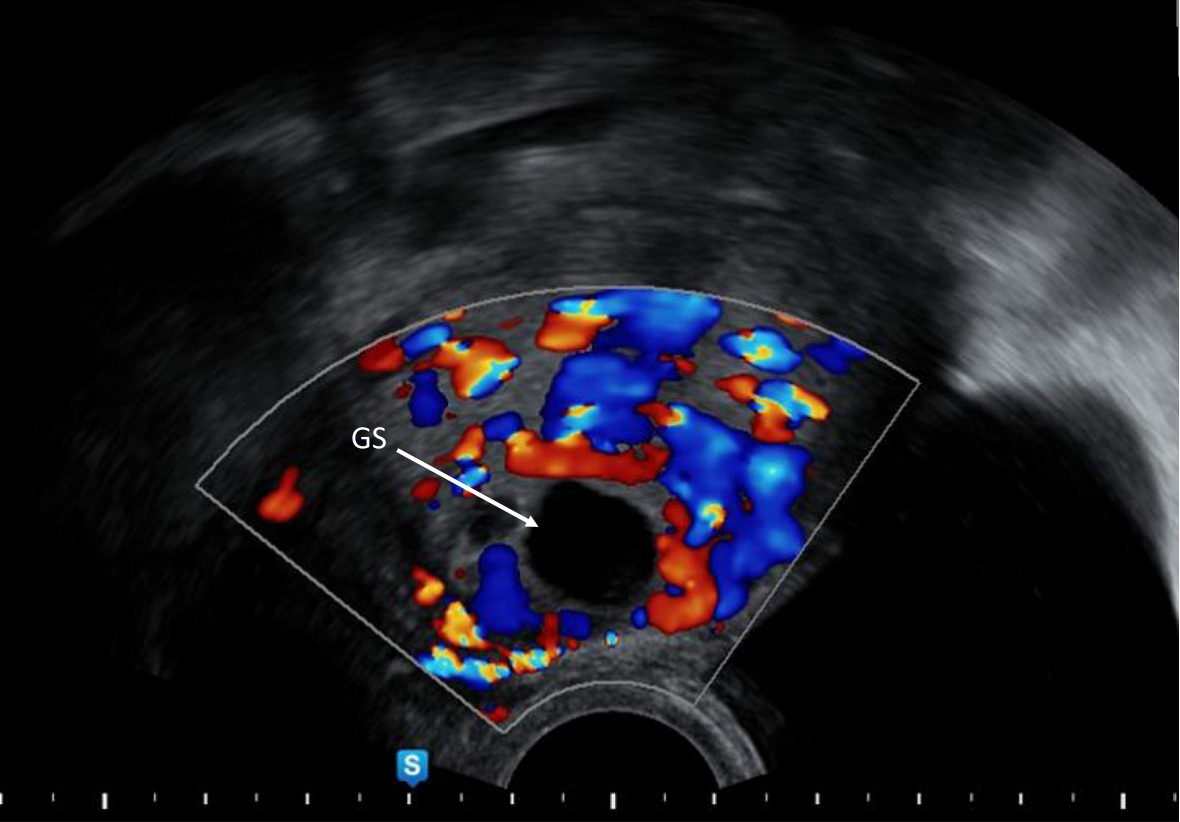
Sonographic diagnosis of caesarean section scar pregnancy in the early first trimester. Peritrophoblastic vascularization on ultrasound Doppler. GS, gestational sac
